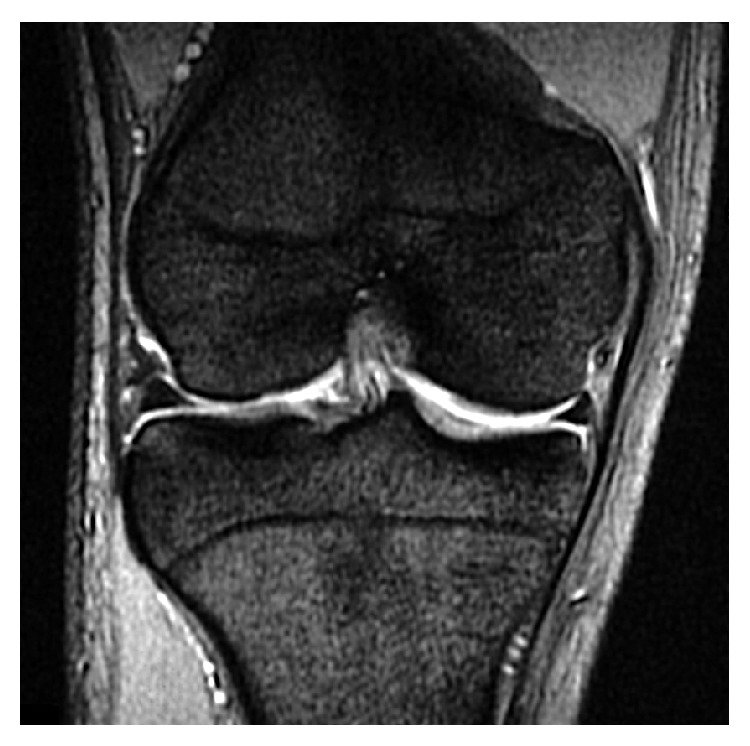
T2-weighted coronal magnetic resonance image of the right knee. Small triangular fragment located over the normal lateral meniscus.
